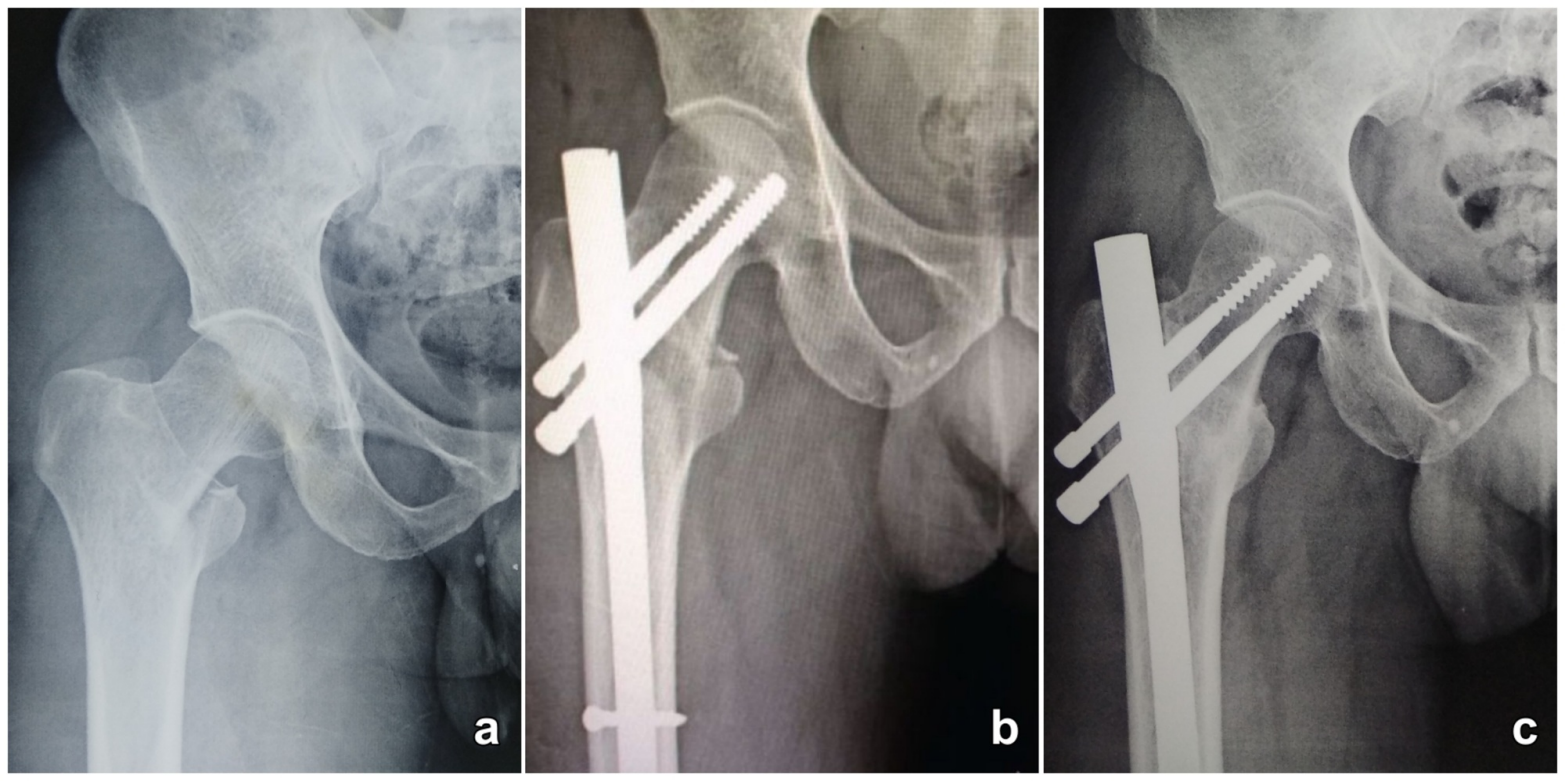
Radiograph of 45-year-old male with 31A1 intertrochanteric fracture fixed with proximal femur nail. (a) Pre-operative radiograph showing two-part fracture. (b) Radiograph taken on day 1 post-surgery. (c) Radiograph taken six months post-surgery showing complete union of fracture.
